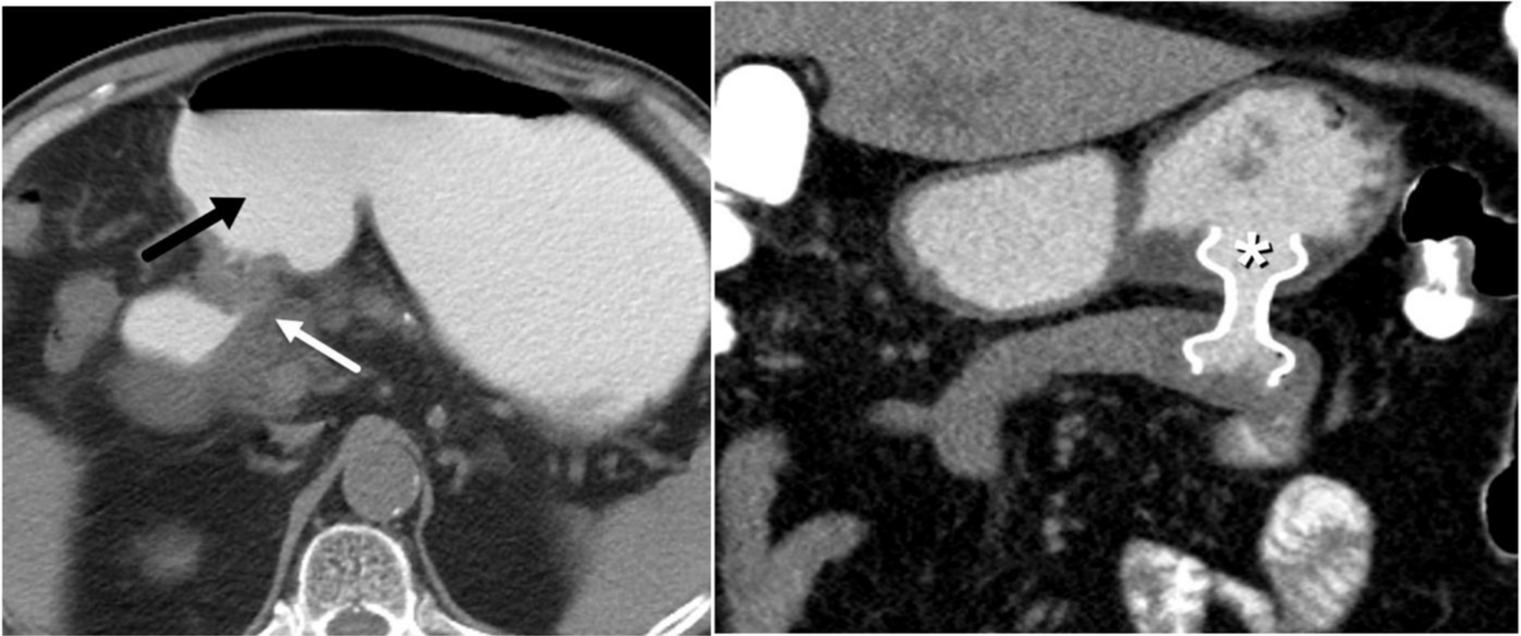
83 year-old male with pancreatic carcinoma receiving palliative endoscopic gastrojejunostomy. A. Contrast-enhanced axial CT image showing partial gastric outlet obstruction with distended stomach filled with oral contrast (black arrow) due to known pancreatic carcinoma (white arrow) at pylorus and first portion of duodenum. B. Contrast-enhanced coronal CT image shows palliative gastrojejunostomy via LAMS (white asterisk) demonstrating luminal patency that relieved obstruction and prevented need for surgery
